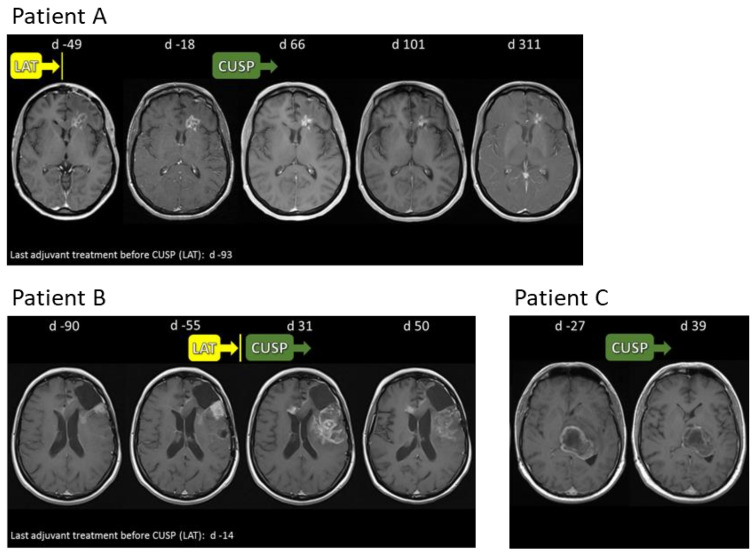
Representative T1-weighted MRIs of patients (A–C) after application of contrast media. LAT: Last adjuvant treatment before initiation of CUSP.
